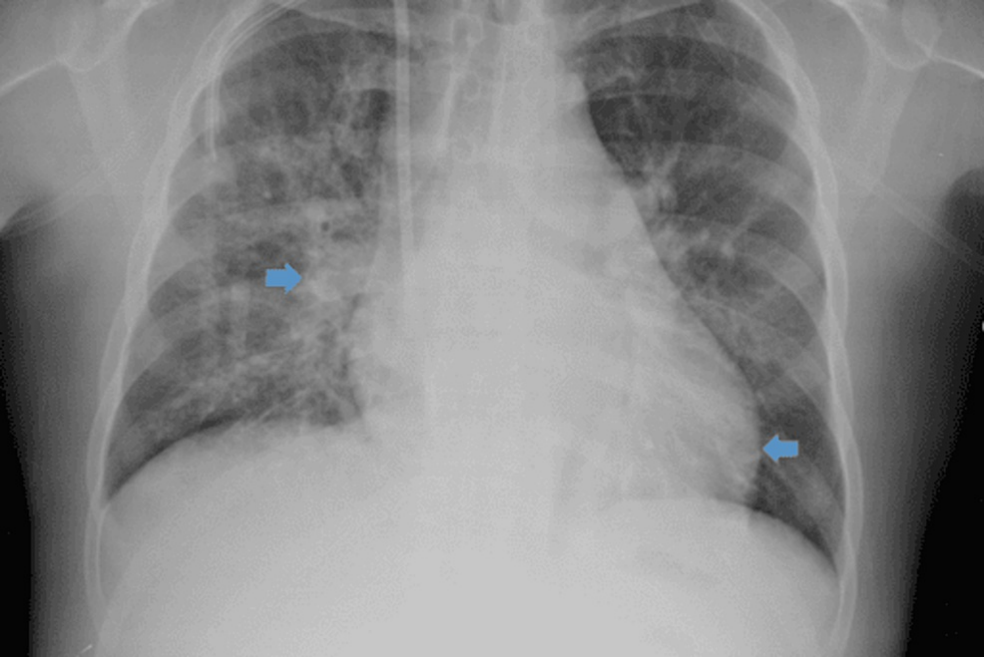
Chest X-ray on admission Chest X-ray reveals mild cardiomegaly and right perihilar edema.
